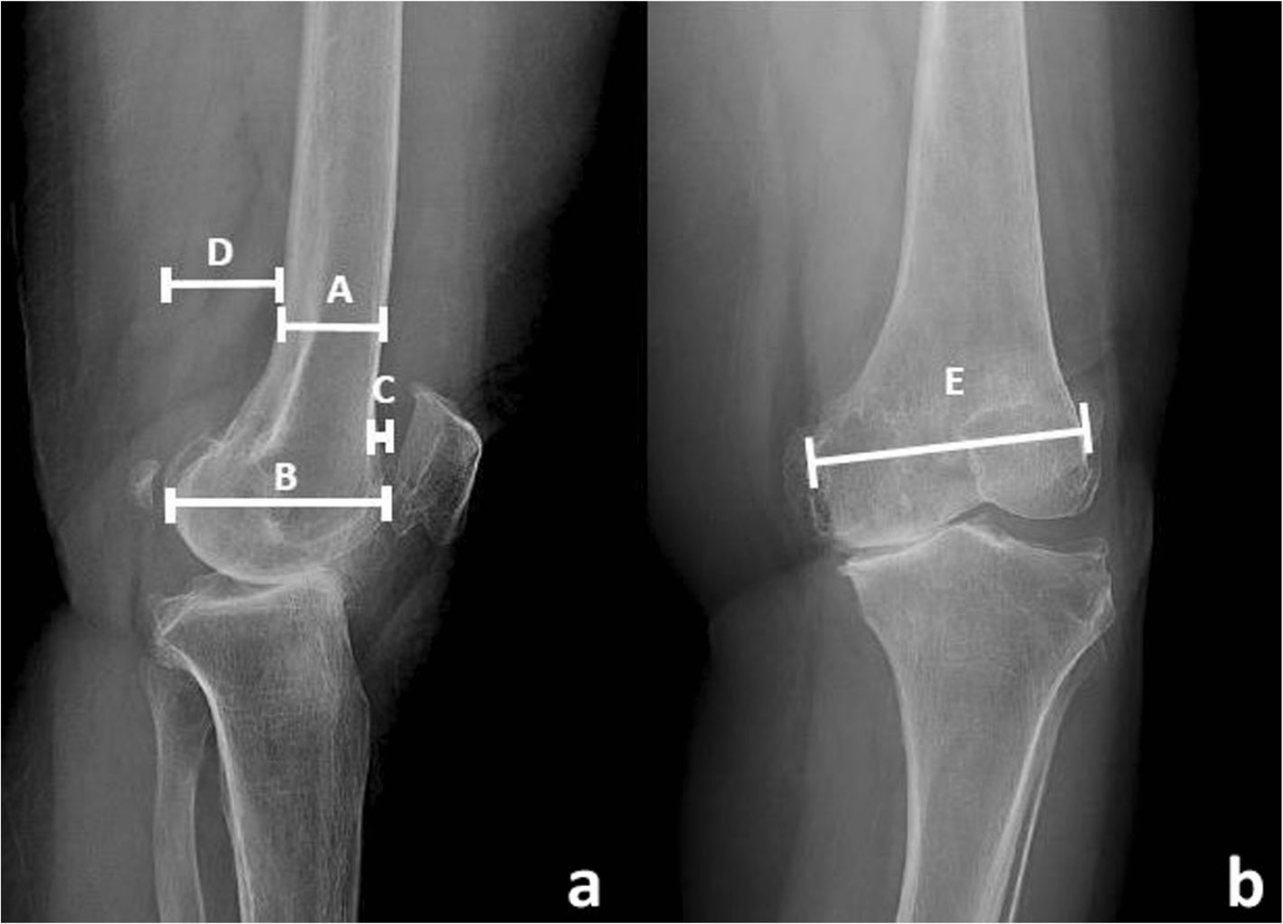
Preoperative radiographic measurements of distal femoral morphology. a: Measurement technique of diameter of AP femoral canal (A), diameter of AP femoral condyle (B), anterior femoral offset (C) and posterior femoral offset (D) in lateral view. b: Measurement technique of ML diameter of femoral condyle in AP view (E)
